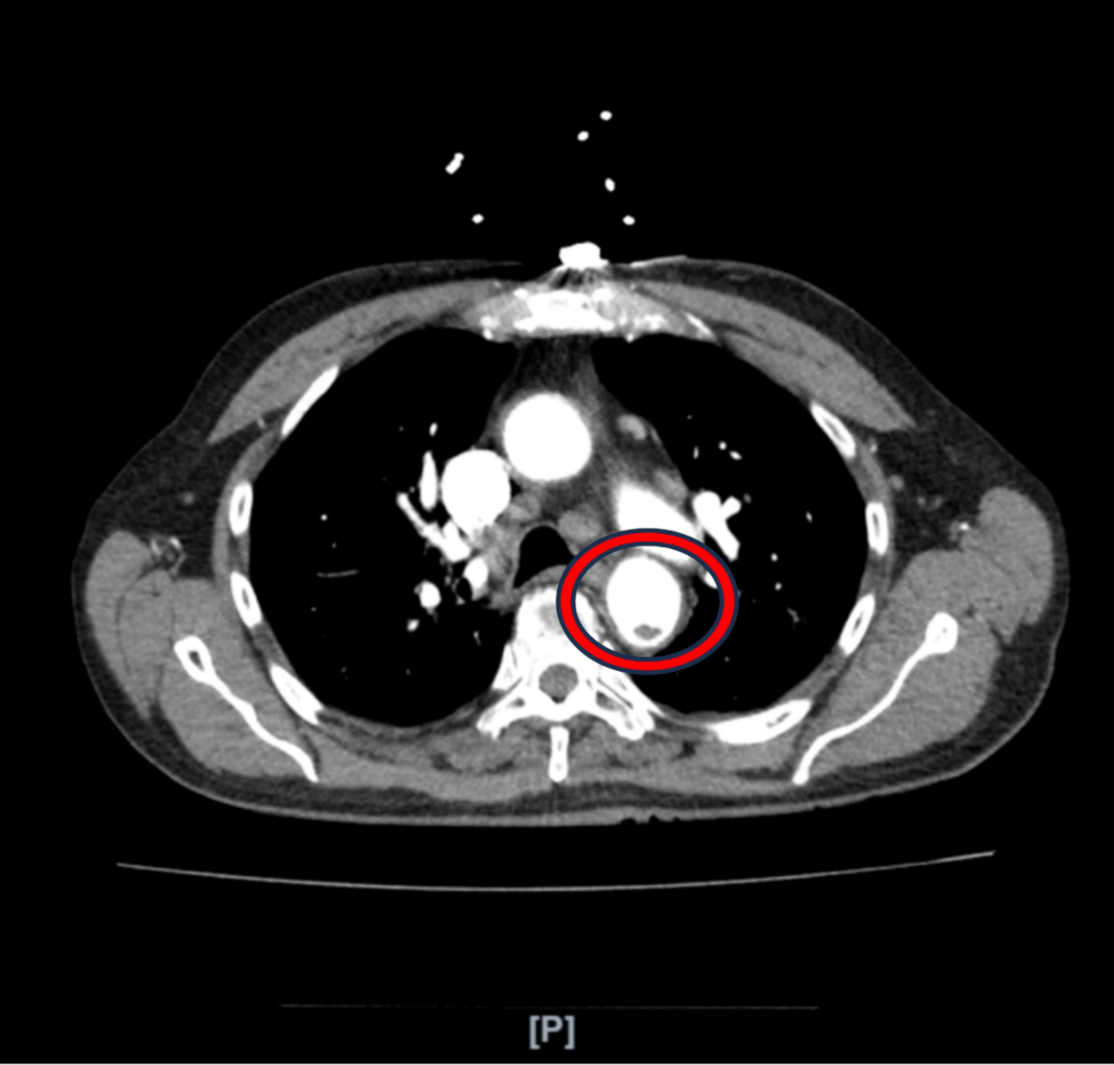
Transverse CT cut revealing a filling defect on the descending aorta The filling defect likely represents AAD. Mild pericardium effusion was also noted.
